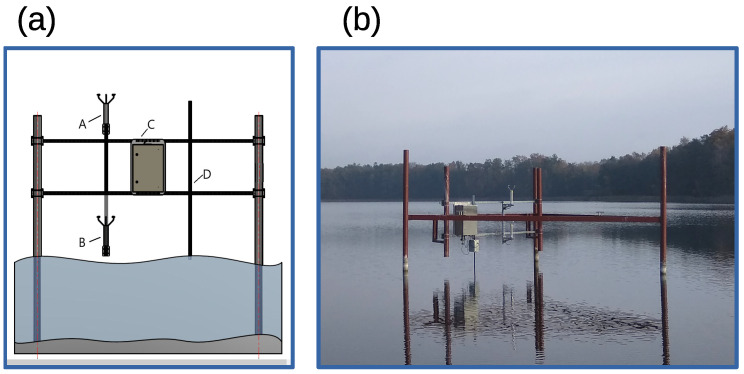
Measurement device: (a) design concept: A,B—ultrasonic anemometer, C—measurement box, D—wave sensor; (b) device mounted at the test point.
